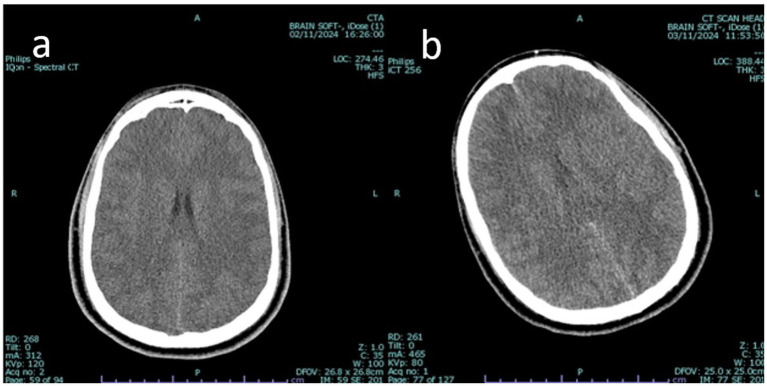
Two slides of axial brain computed tomography of Case 3. (a) Diffuse cerebral edema with effacement of the ventricles. (b) Worsening of the diffuse cerebral edema with complete effacement of the ventricles.
